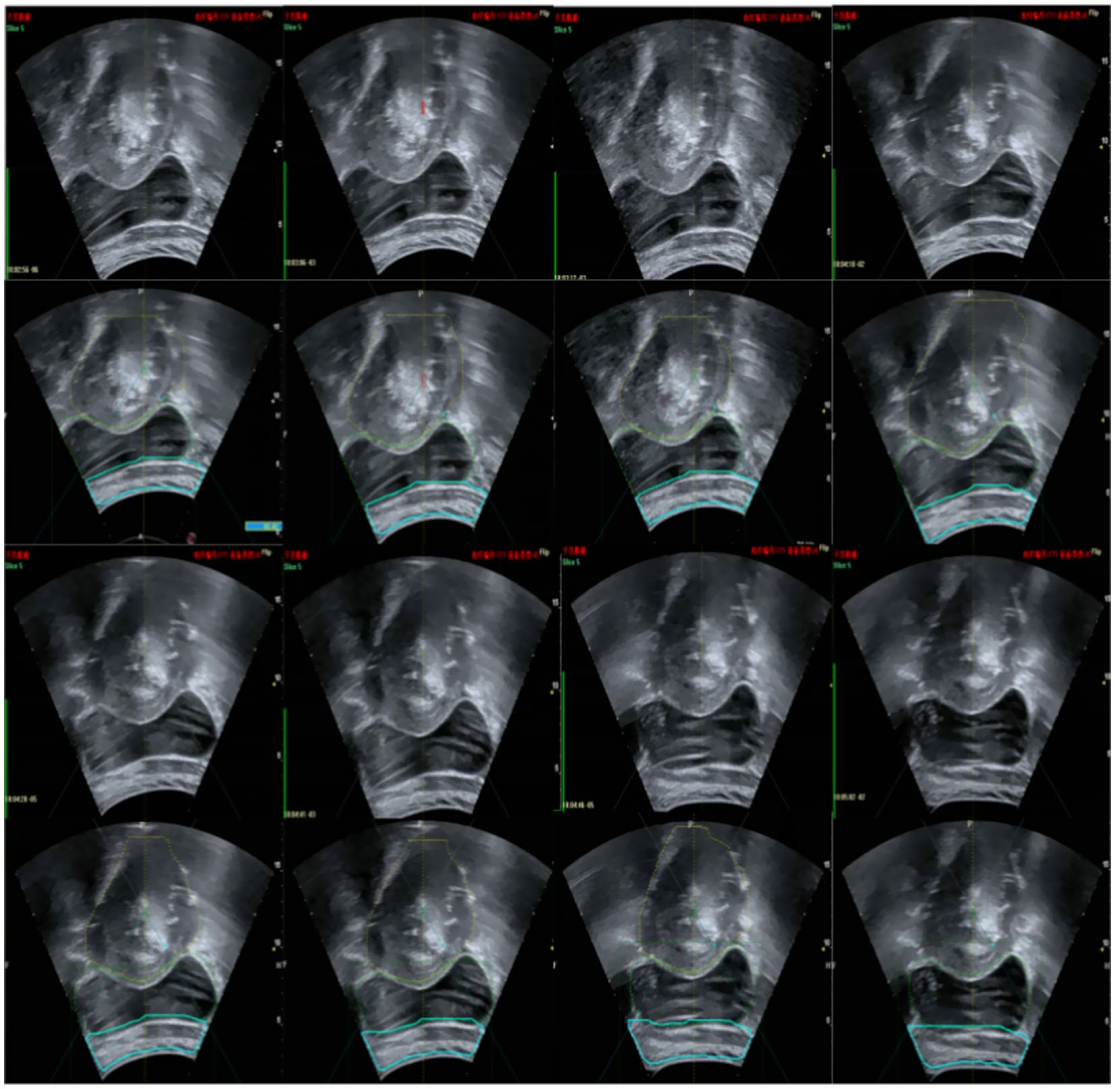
Ultrasonic video stream tracking image.
